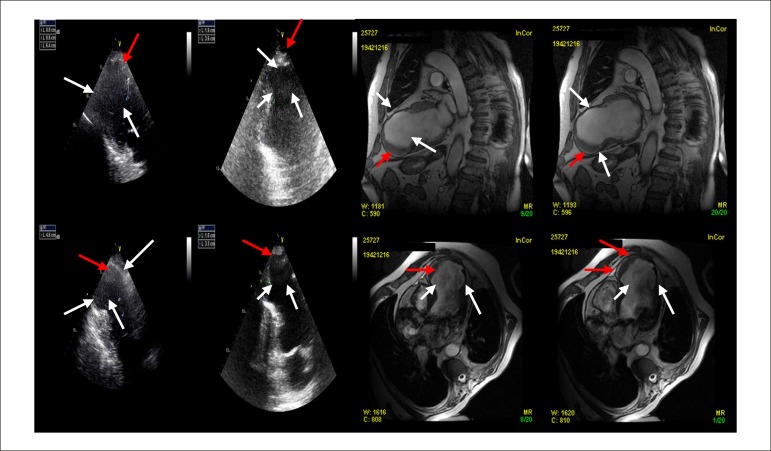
Left panel: Transthoracic echocardiogram showing dilated left chambers
